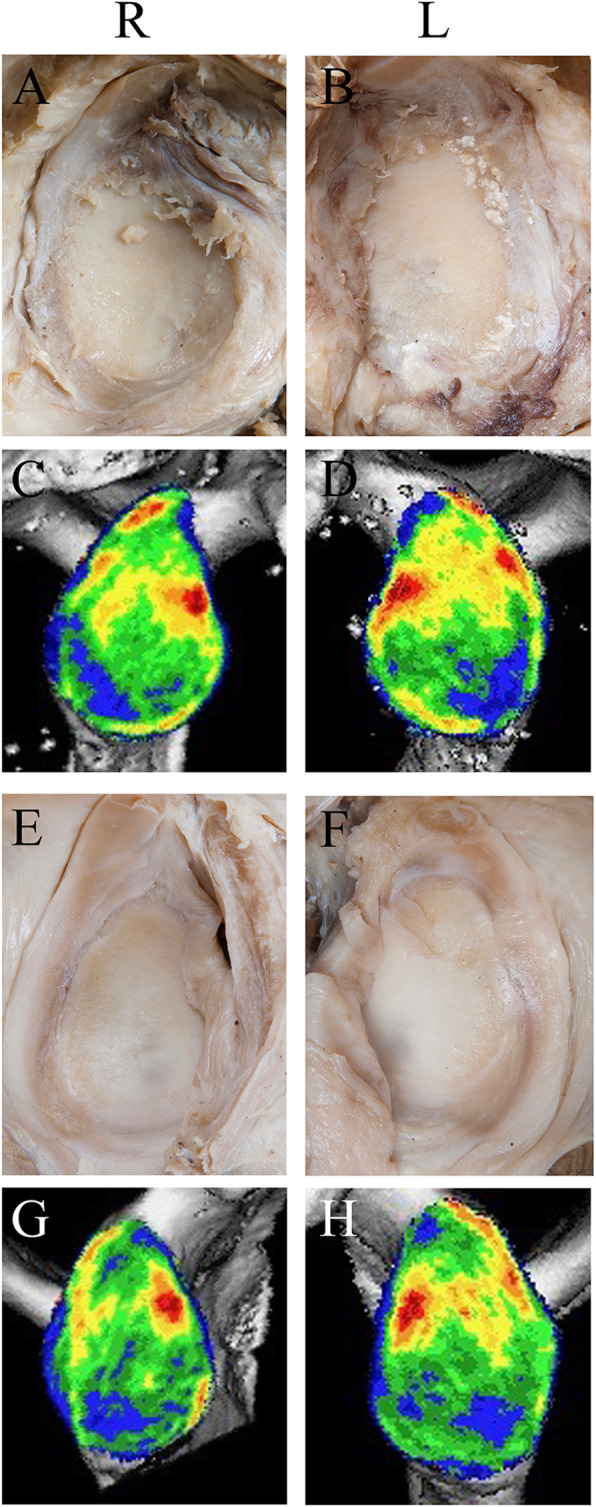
Mineralisation distribution in glenoids with inclination angles < 10°. A-D 73 years old male (inclination: R 7.8°, L 3.3°; retroversion: R -6.9°, L -6.7°). C,D On both sides, in addition to anterior mineralisation, density maxima in cranial and dorsal quadrants corresponding to cartilage defects (A,B). Cartilage thinning in the anterior, more caudal quadrants (A,B) mirrored by CT-OAM (C, D). Some spots along the glenoid rim, probably osteophytes. E-H 72 years old male (inclination: R 7.6°, L 2.9°; retroversion R -1.7°, L − 0.4°). In the left shoulder, note higher mineralisation in cranial quadrants (H) and cartilage lesion (F). Some spots along the glenoid rim probably osteophytes
